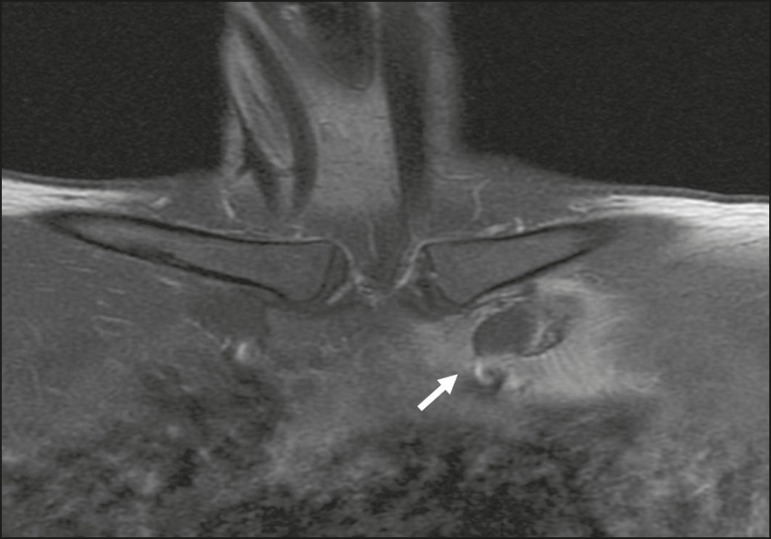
A 38-year-old female with chest wall pain. An MRI scan shows edema
